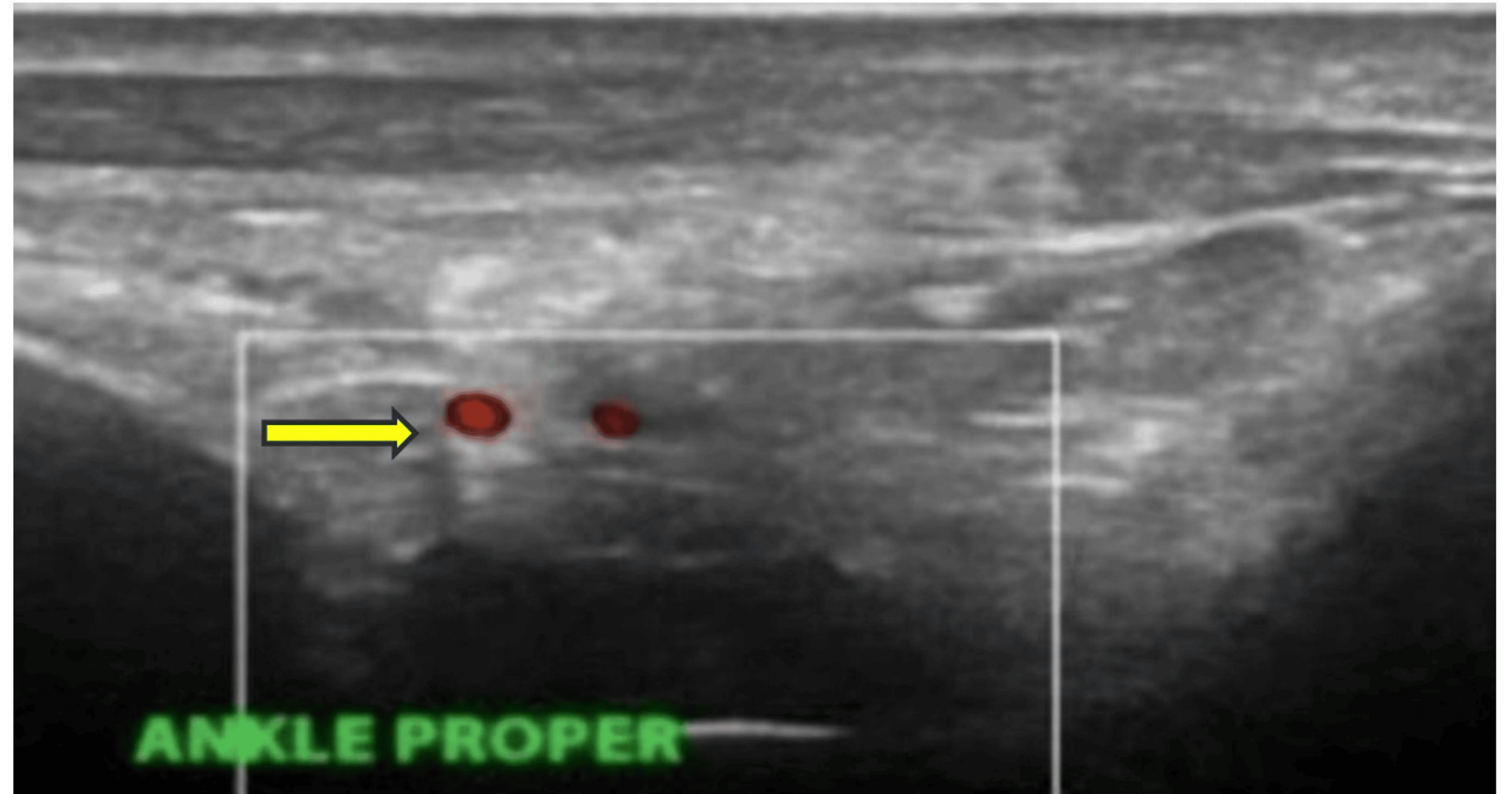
Ultrasound findings of the left ankle anterior longitudinal view showing synovial hypertrophy (yellow arrow) with a grade 2 Doppler signal
